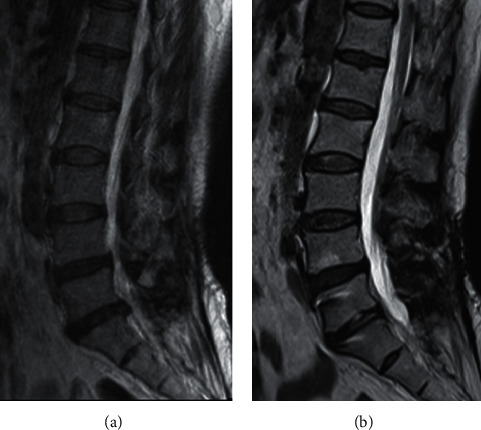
A case of a 53 years old female patient with RLDH at L5-S1. MRI scans showed rehydration at 22-month follow-up after transpedicular dynamic stabilization.
